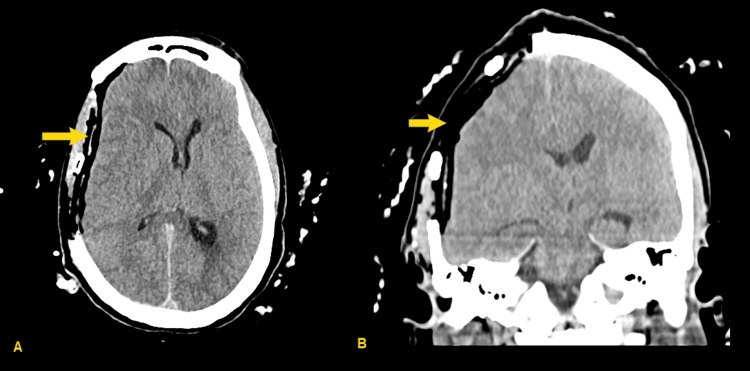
Post-hemicraniectomy CT head, with resolution of previously demonstrated subdural hematoma (SDH) (yellow arrows) (A) Axial image, (B) Coronal image
